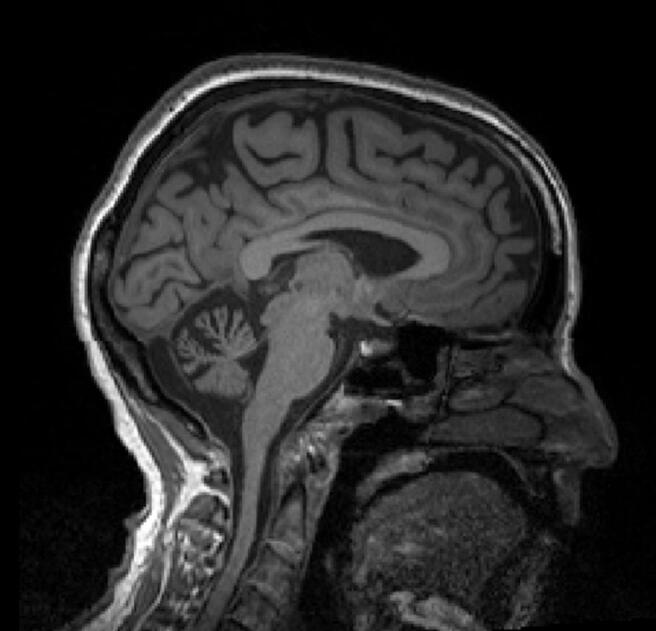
Sagittal cMRI (FLAIR sequence) as an example at measurement time T0.1.
